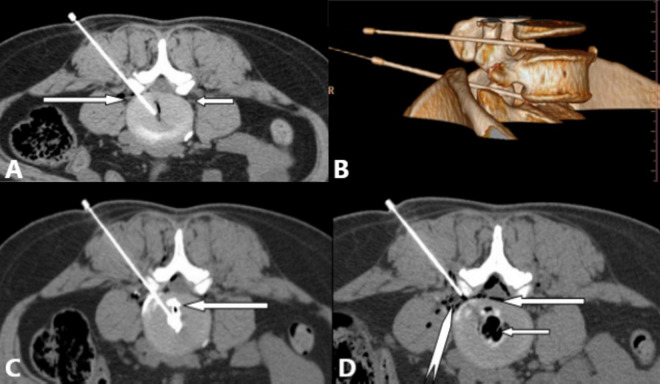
Multi-slice spiral CT provides high-resolution, non-overlapping axial images for precise puncture; offers multiplanar reconstruction (MPR) and volume rendering (VR) capabilities for rapid, accurate guidance; and allows evaluation of lumbar annular fissures and oxygen-ozone distribution. (A) Right posterolateral approach for L4/5 intradiscal treatment. White long arrow: left L5 nerve root; white short arrow: right L5 nerve root. (B) VR 3D reconstruction showing puncture paths (safe triangle area) for L4/5 (white short arrow), and L5/S1 (white long arrow) discs. (C) L4/5 discography revealing single lumbar annular fissures (white long arrow) and contrast agent distribution, guiding treatment planning. (D) Needle position for epidural anterior space treatment post-intradiscal therapy at L4/5. White long arrow: epidural oxygen-ozone distribution; white short arrow: intradiscal oxygen-ozone distribution; white dovetail arrow: L5 nerve root.
